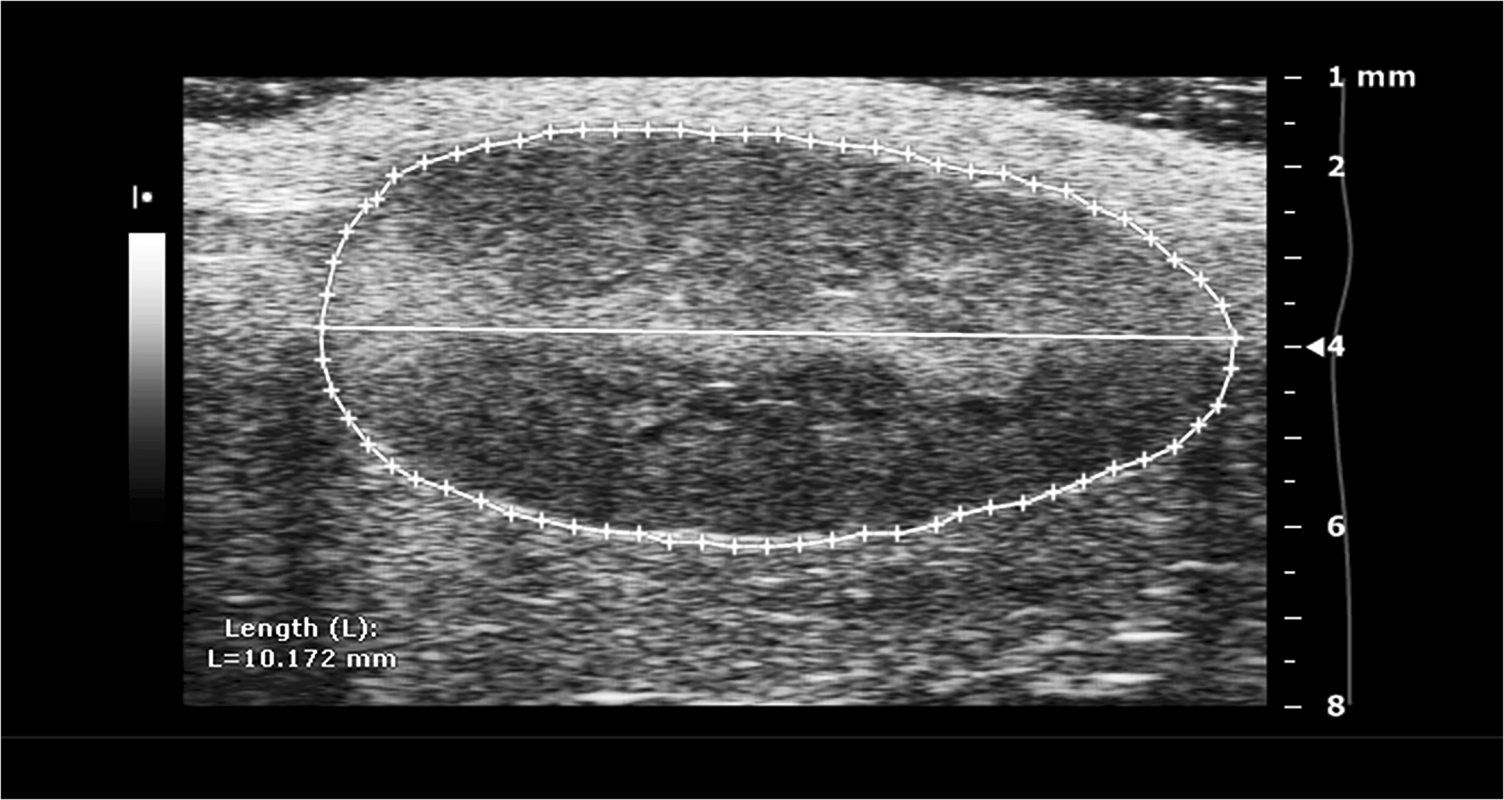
B-mode ultrasound measurement of kidney length in long-axis view. Off-line measurements of kidney length.
